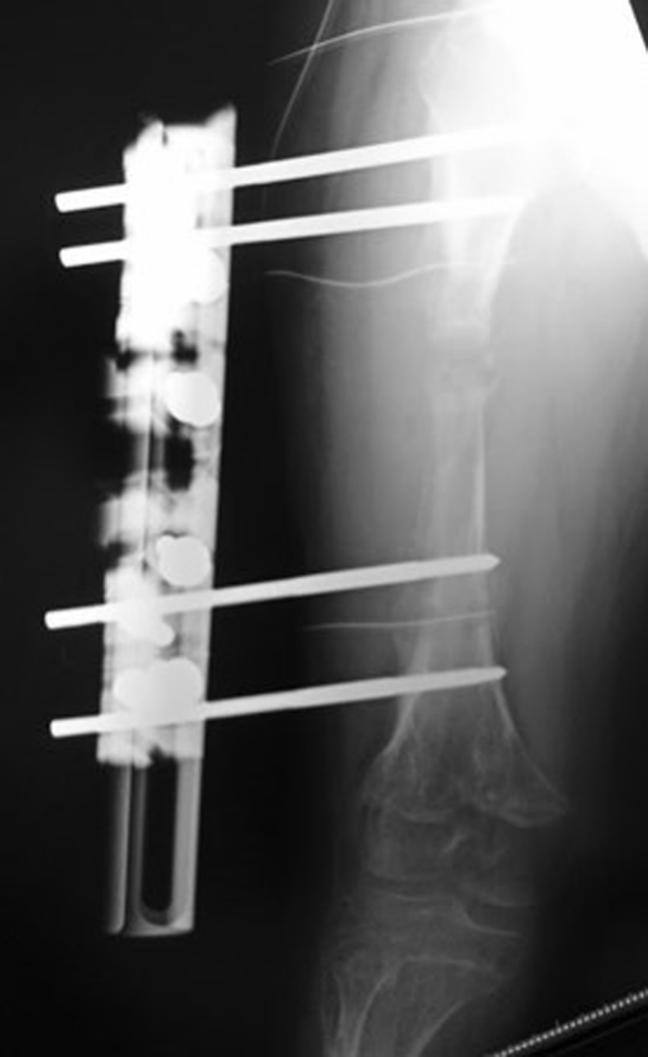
X-ray shows deep pin-tract infection 5 months after the initial surgery
